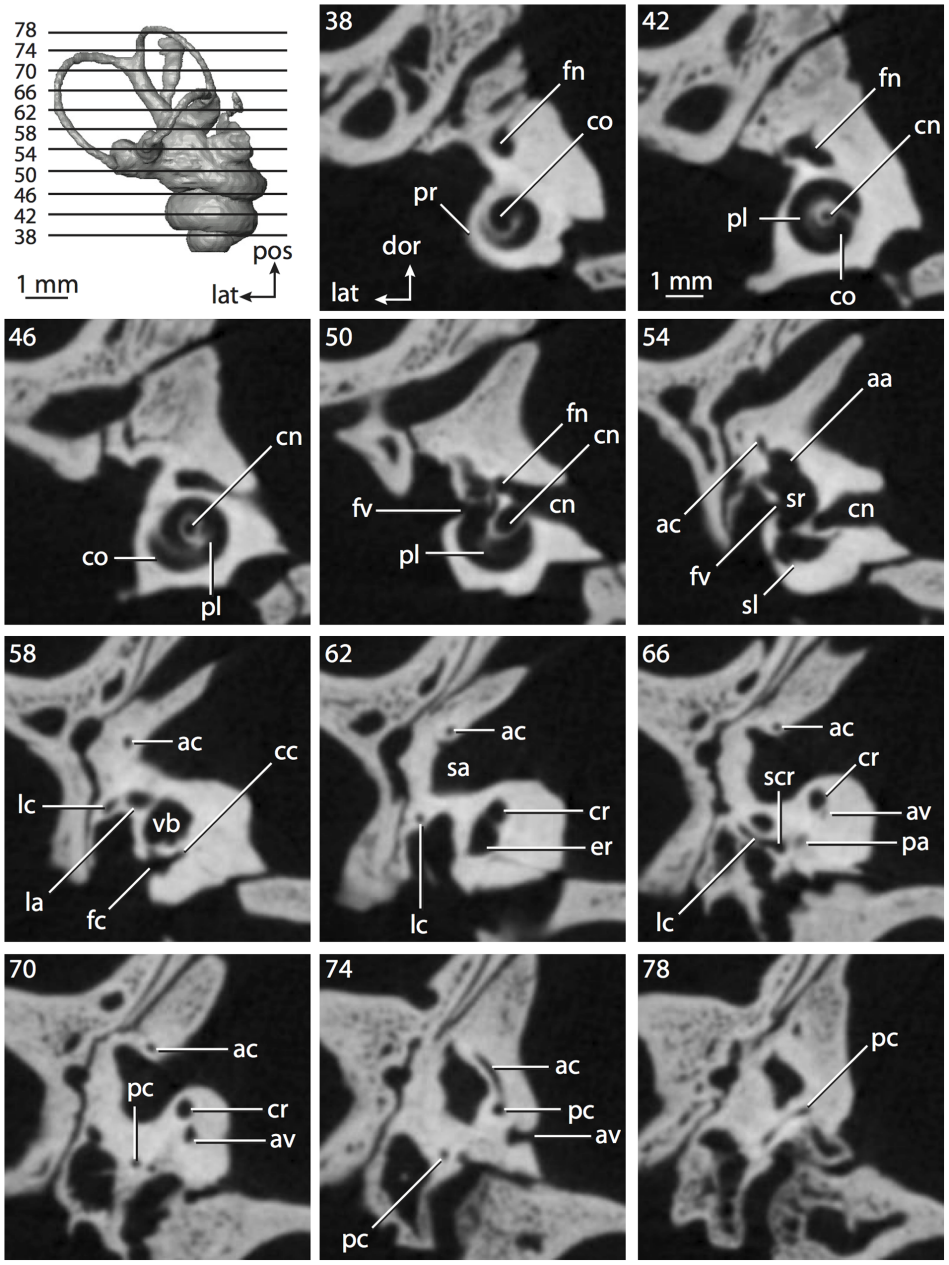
Original CT slices through ear region of*Didelphis virginiana.* Numbers refer to specific CT slices. Abbreviations listed at the end of the Materials and Methods section.
